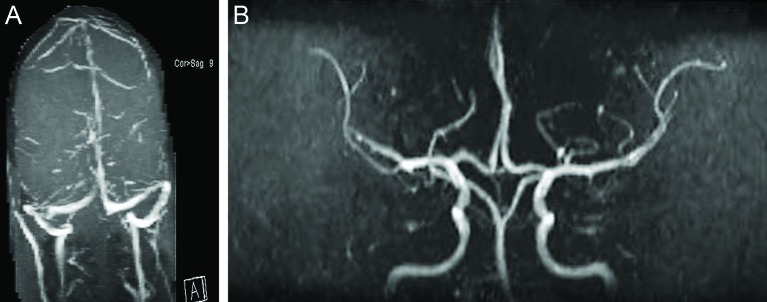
(A and B) Follow-up brain MRI and MRV after 6 months. Normal MRI and MRV of the brain with no defects in the superior sagittal, right transverse and sigmoid sinuses, and no acute intracranial haemorrhages.
